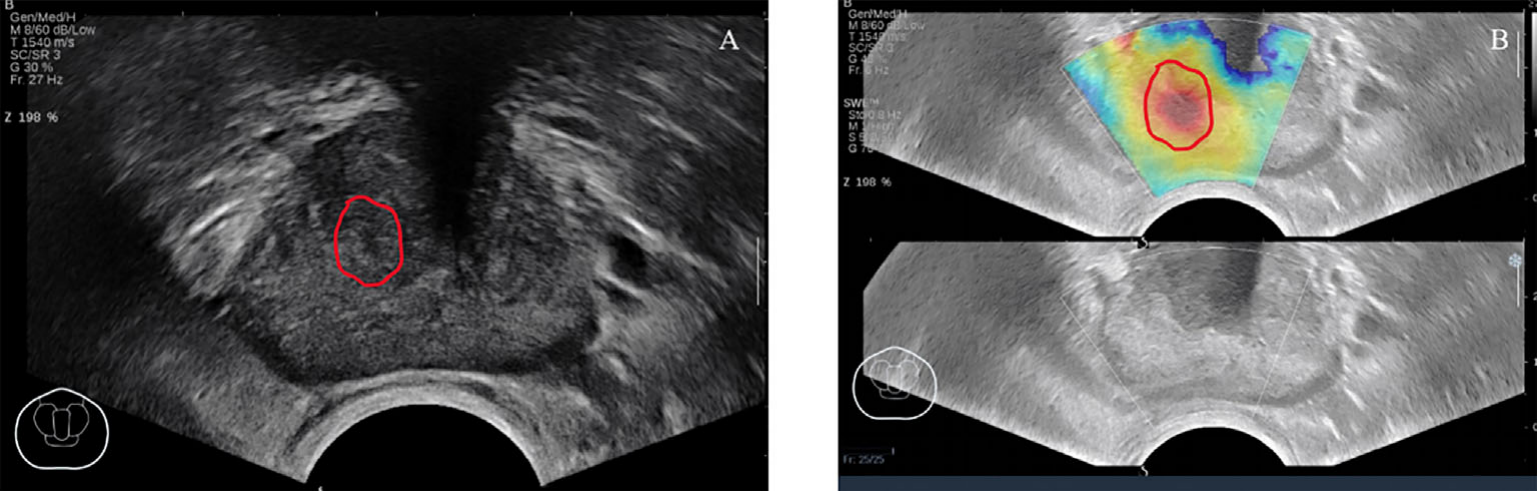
A 67-year-old patient had no obvious abnormal lesions in B-mode ultrasound (A), SWE (B) showed that the local tissue became stiff, and the biopsy result was Gleason = 3 + 4. ROI was delineated under the guidance of the abnormal area of SWE.
